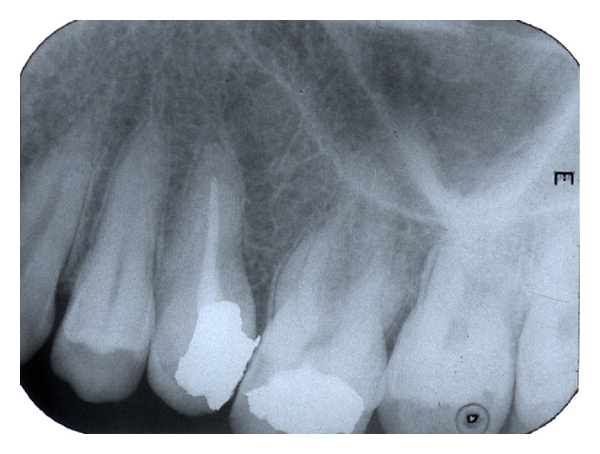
Periapical radiograph taken from the symmetrical region of the maxilla shows no unusual morphology of the teeth.
